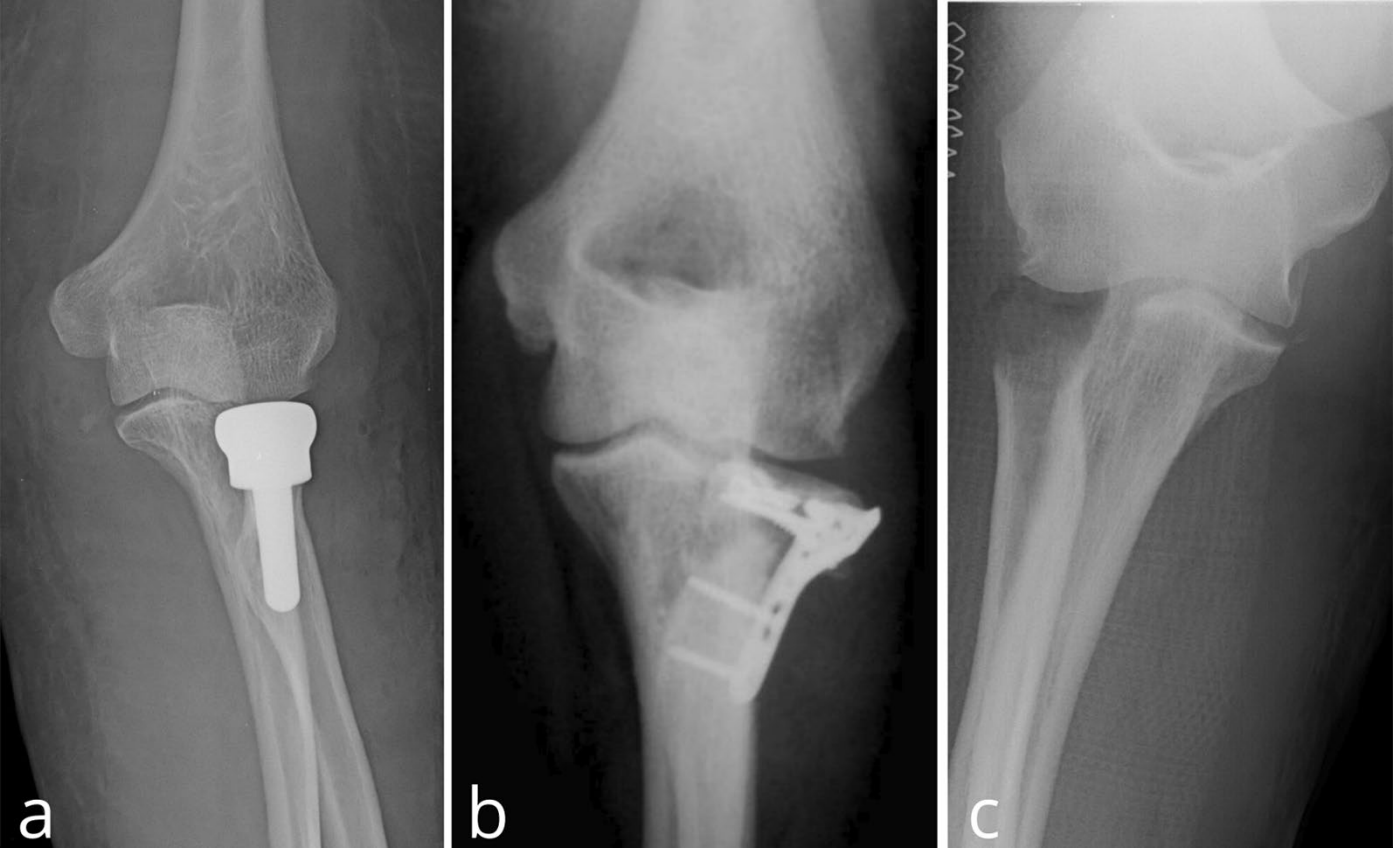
Postoperative anteroposterior X-ray of Mason type III radial head fracture treated with prosthetic replacement (a), open reduction and internal fixation (b) and resection (c)
